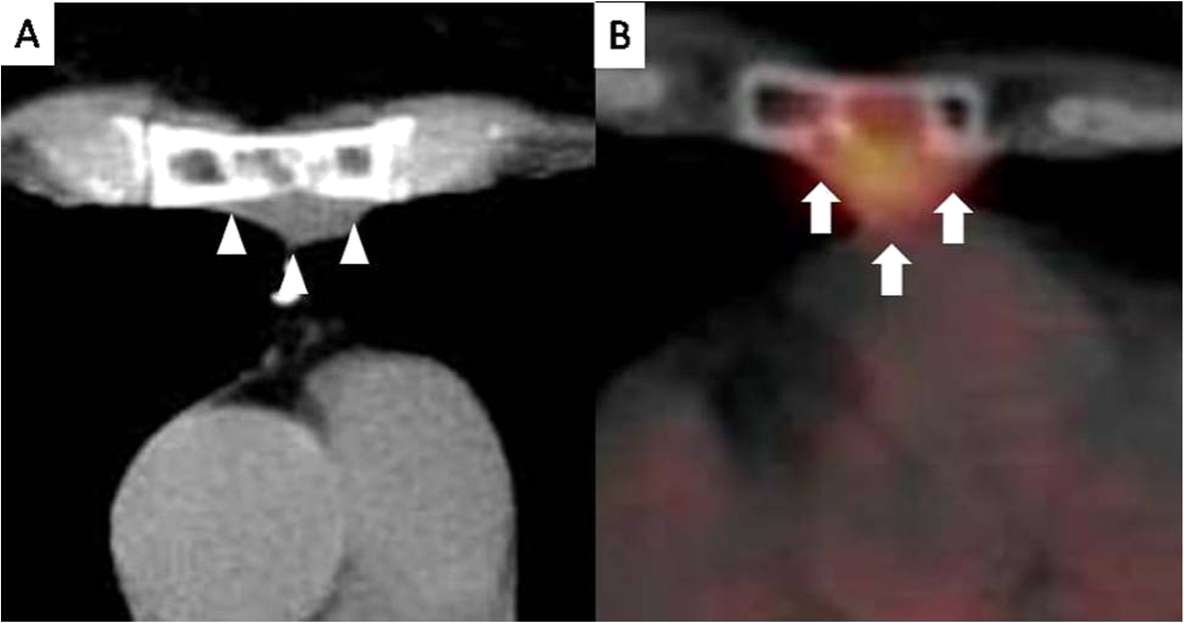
The finding of chest CT. (A) Chest computed tomography showed a round, well-defined, low-density nodule measuring 3.3 x 2.8 cm (arrows), which was located at almost the center of the sternum with bone lysis and erosion. The finding of FDG-PET/CT (B) [^18^ F]Fluorodeoxyglucose positron emission tomography/computed tomography demonstrated fluorodeoxyglucose accumulation (arrowheads) in the tumor. The standardized uptake value was 5.51.p.
